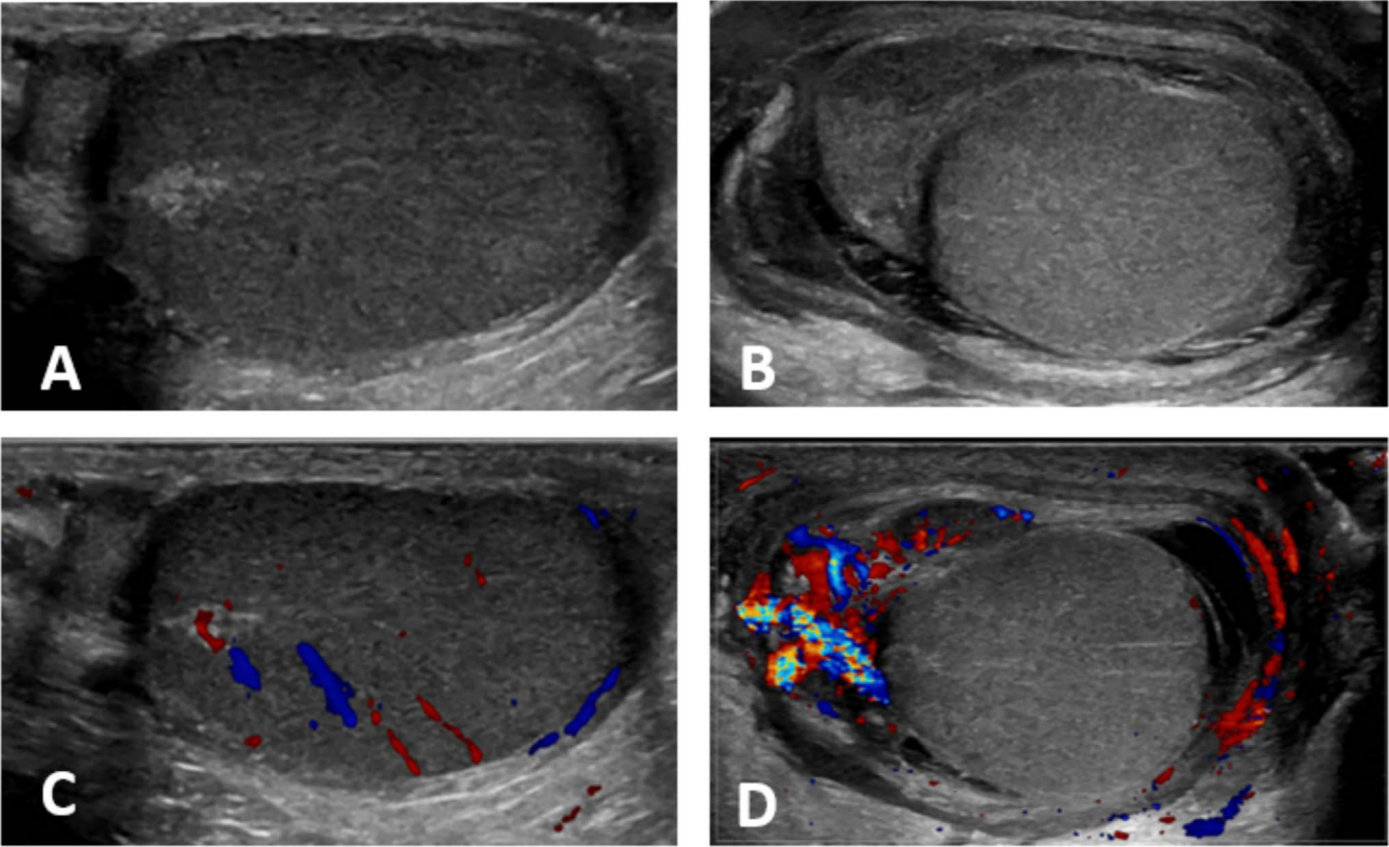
Representative ultrasonography images of epididymitis. Compared to the left side in A and C, the right epididymis in B and D is enlarged and shows increased blood flow. Additionally, decreased blood flow is observed in the right testis
